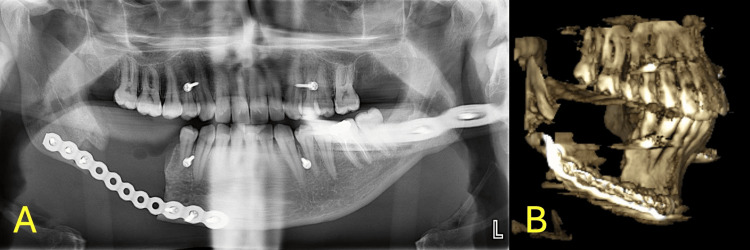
Post-operative radiographic images A. Post-operative panoramic radiograph. B. Post-operative 3-dimensional computed tomography.
